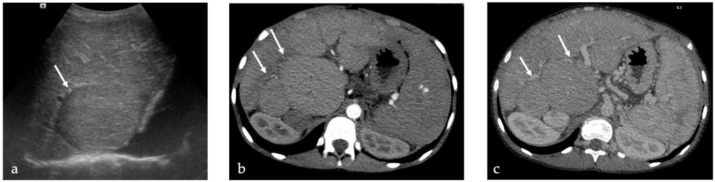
Macroregenerative nodules in a 14-years old boy with biliary atresia. US (a) with large isoechoic nodules with no vascularization on Doppler. CT with arterial (b) and venous (c) acquisition: multiple diffuse isodense iso-enhancing nodules.
